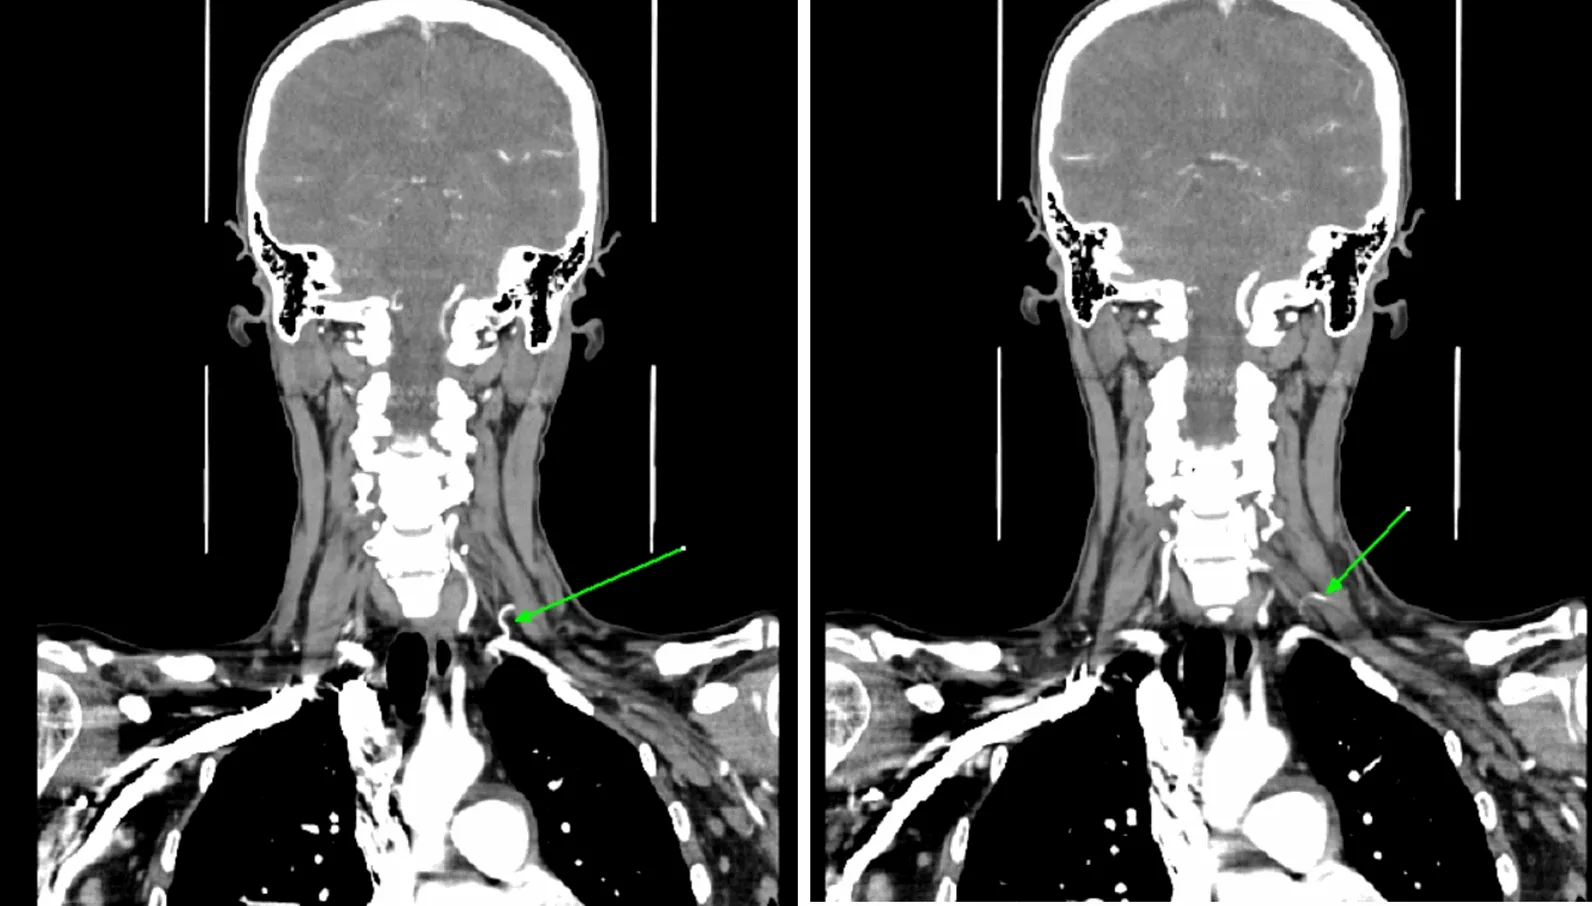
Coronal reconstruction of computed tomography angiogram acquired after the injections showing a large prominent left DSA, originating directly from the first part of the subclavian artery and taking a tortuous course into the interscalene space
